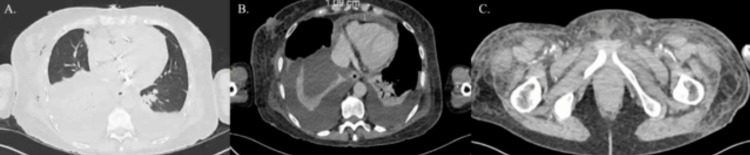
Thoracoabdominal computed tomography demonstrating pulmonary oedema and pericardial effusion. Axial computed tomography images. A: Diffuse bilateral pulmonary oedema characterized by smooth interlobular septal thickening and dependent alveolar opacities. B: Moderate pericardial effusion surrounding the heart. C: Pelvic soft-tissue oedema with prominent subcutaneous stranding, suggesting systemic inflammatory or edematous changes.
